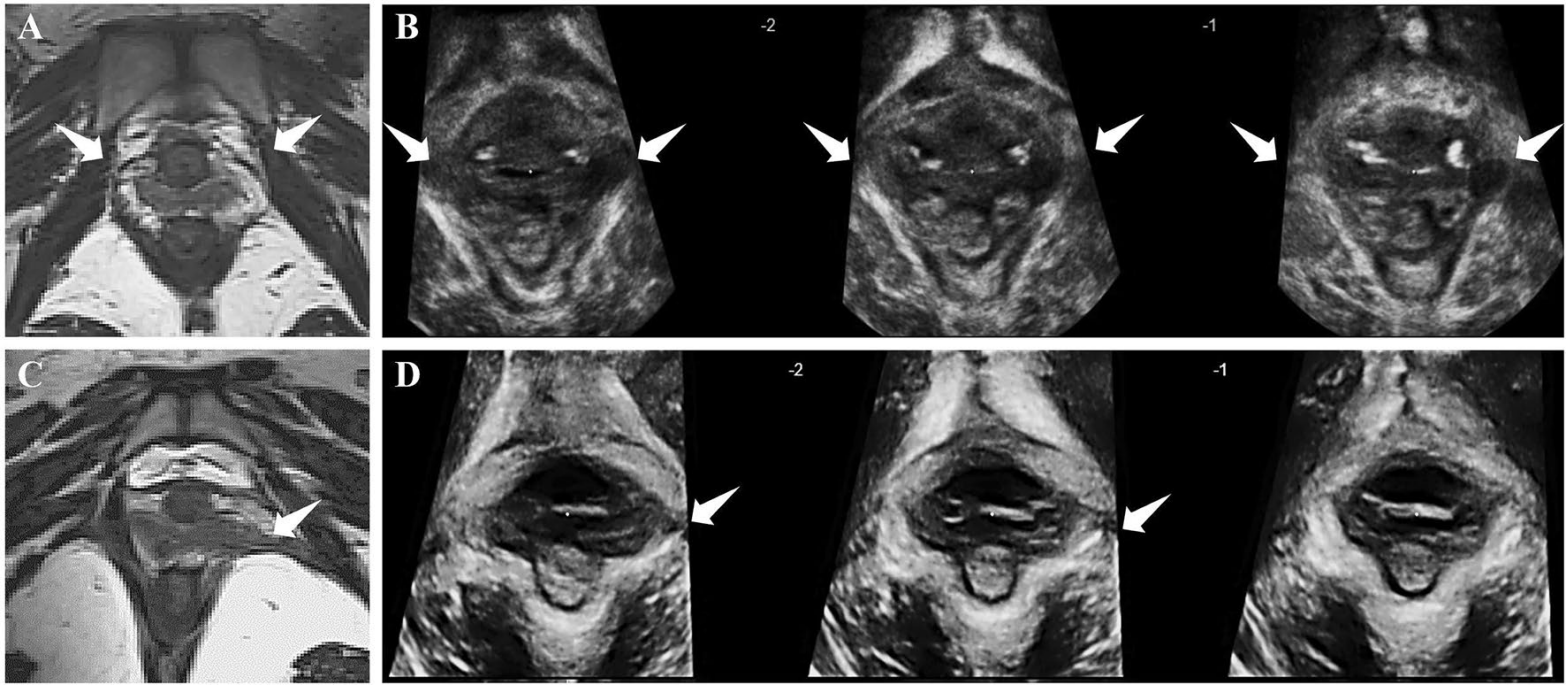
The magnetic resonance image showed the bilateral avulsion (A) and left avulsion (C). The tomographic ultrasound images showed complete avulsions at the both sides on the three central slices (B) and partial avulsion at left side on the three central slices (D). White arrows pointed the positions of the levator ani defect.
